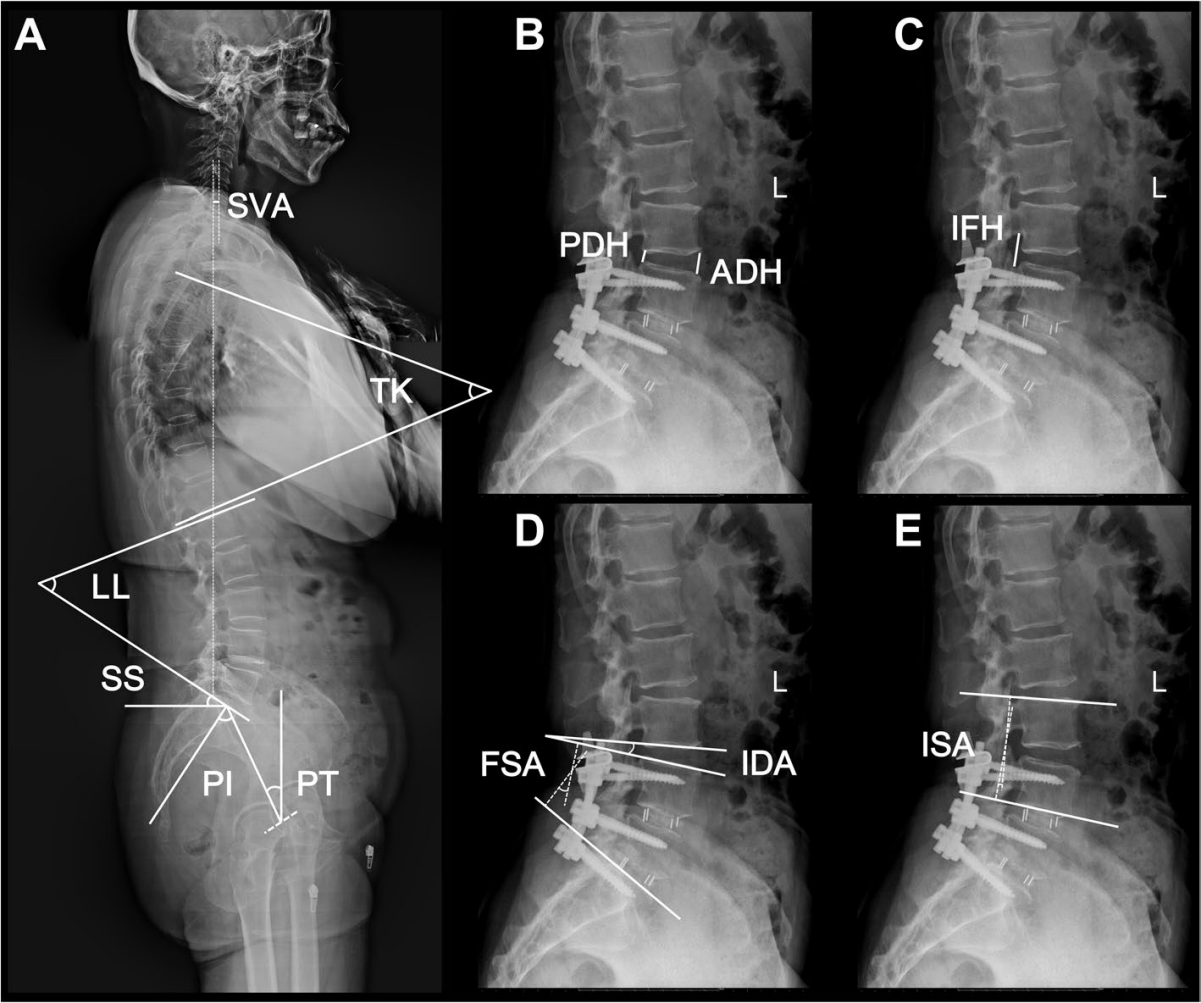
Radiographic evaluation of the spinal sagittal parameters. A SVA indicates sagittal vertical axis, TK indicates thoracic kyphosis, LL indicates lumbar lordosis, SS indicates sacral slope, PI indicates pelvic incidence, PT indicates pelvic tilt. B PDH indicates posterior disc height, ADH indicates anterior disc height. C IFH indicates intervertebral foramen height. D FSA indicates fused segment angle, IDA indicates intervertebral disc angle. E ISA indicates implanted segment angle
